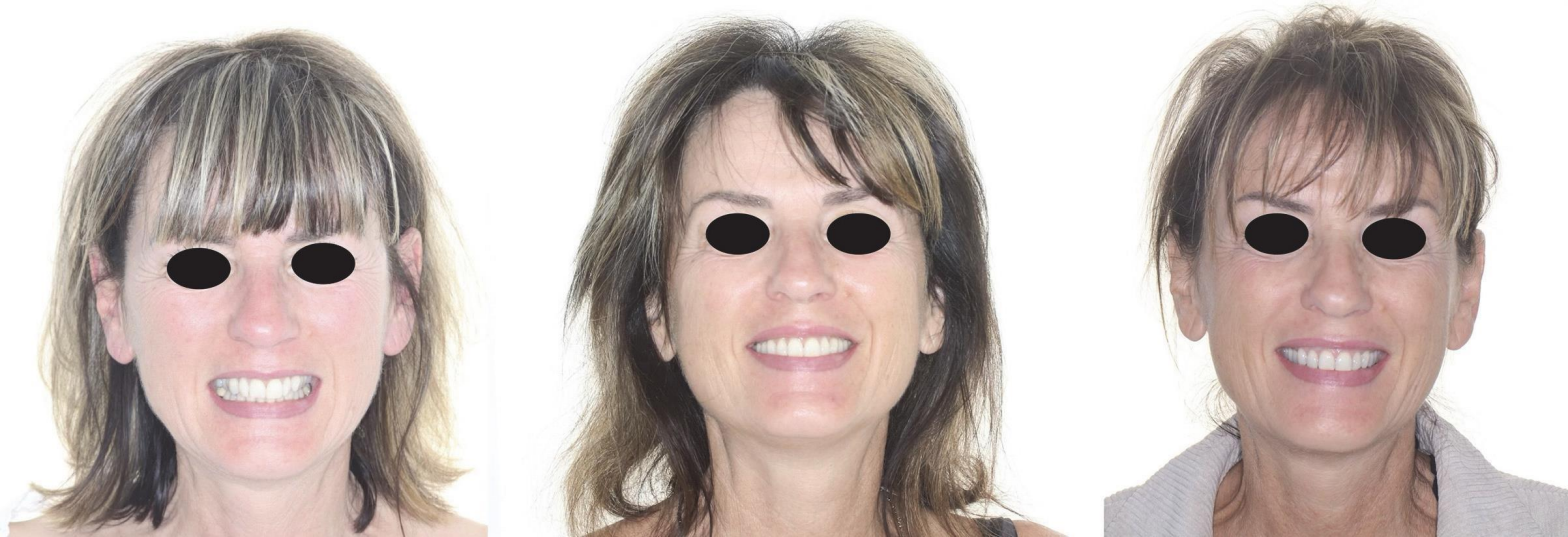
Panoramic radiograph 1‐year posttreatment.
